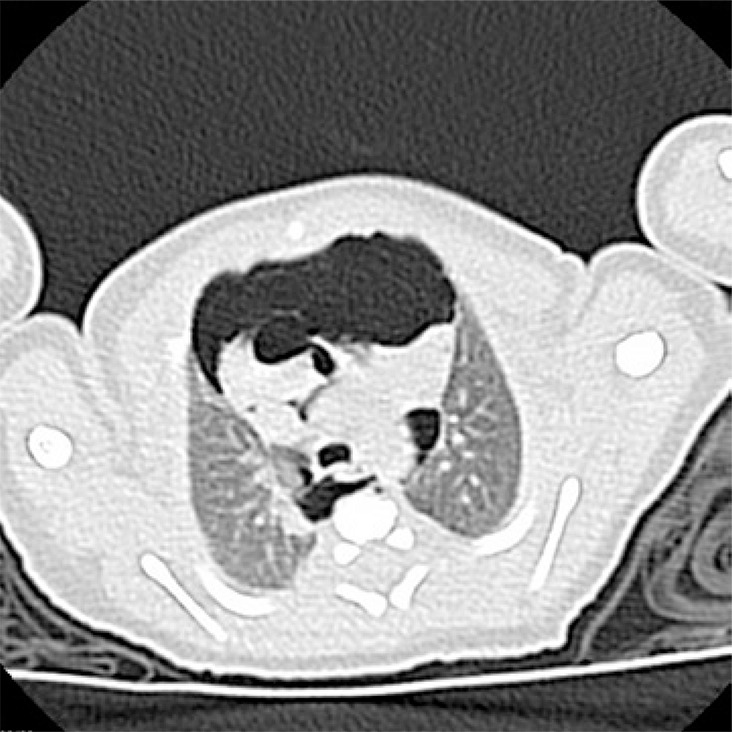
Computed tomography showing an extensive pneumomediastinum with mild septation, unchanged lung parenchyma (cross-sectional cut)
